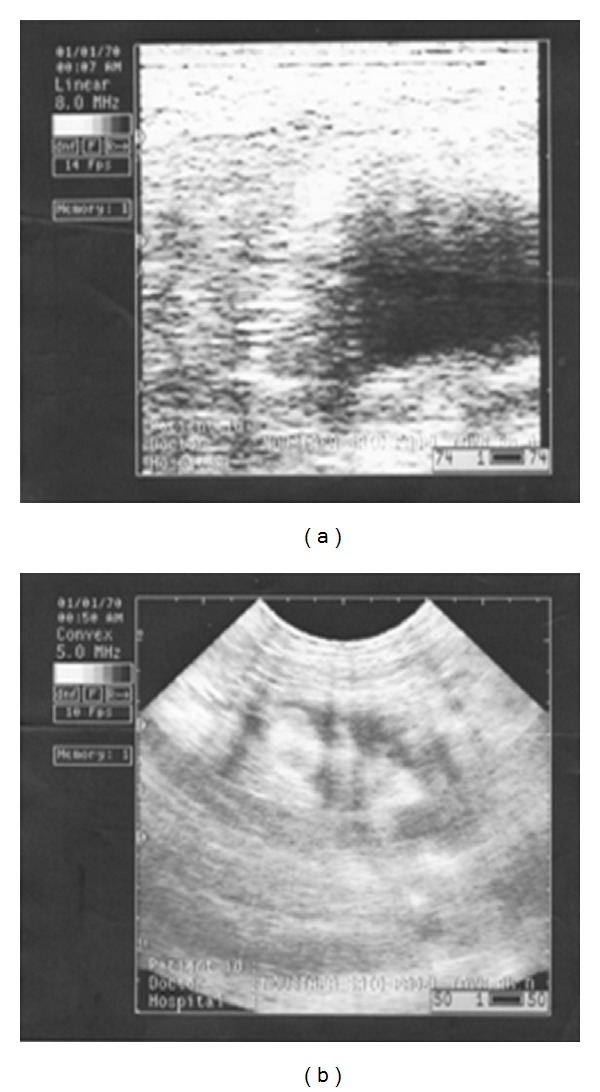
Effect of calcium soap of fatty acid supplementation on pregnancy in ewes by using ultrasonography. (a) Control ewe nontreated was nonpregnant. (b) Calcium-soap-of-fatty-acid-treated ewe was pregnant.
